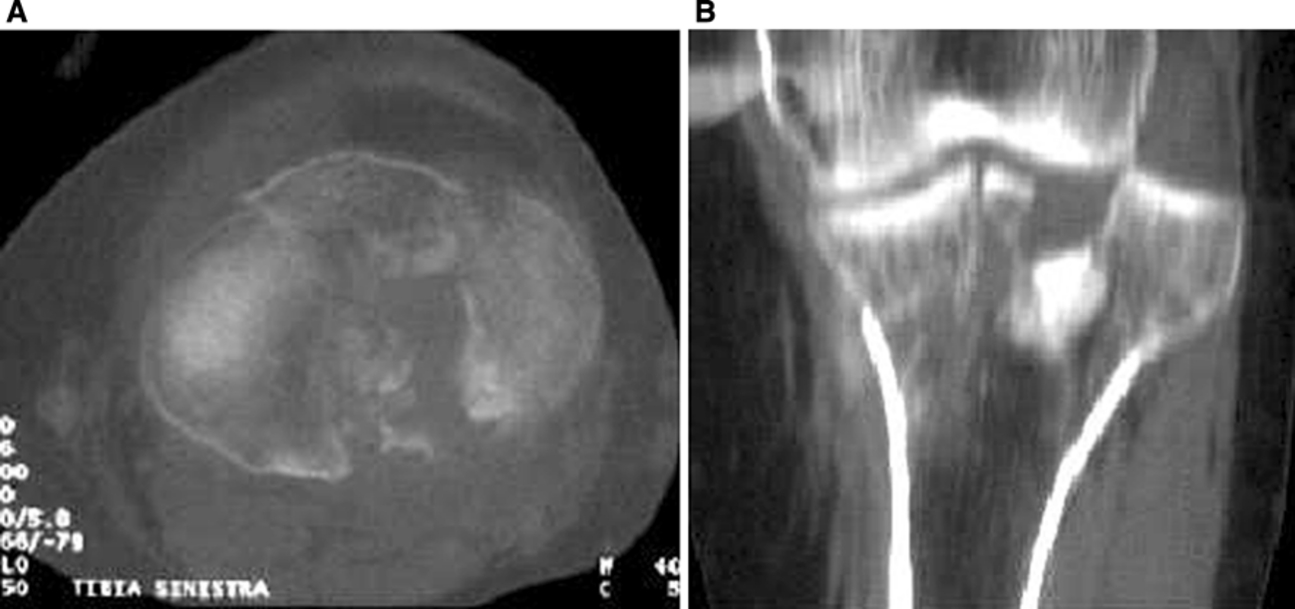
a Schatzker type VI fracture, CT scan. b Schatzker type VI fracture, CT scan
